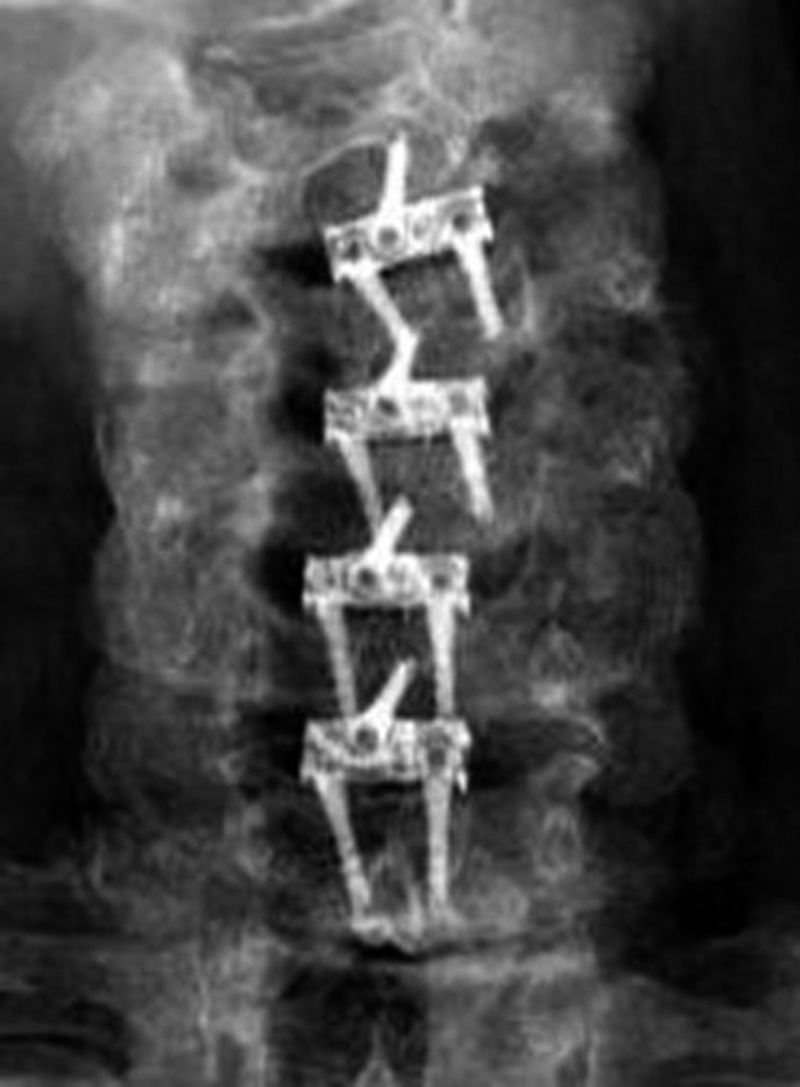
Case Example of a Four-Level ACDF Using the Optio-C Implant AP radiograph at three months after surgery demonstrating preservation of disc heights.
